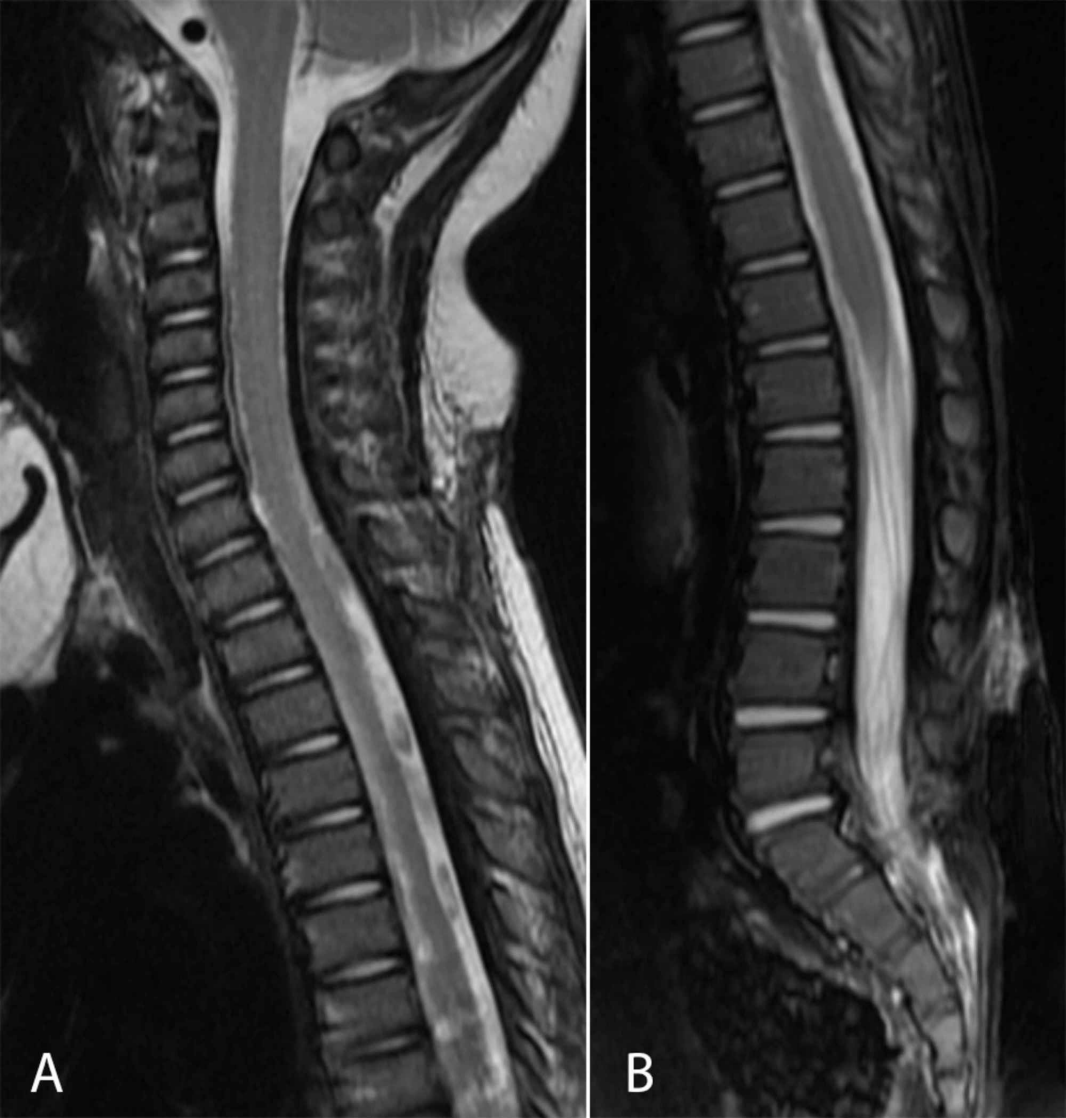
Postoperative T2 MRI of the cervicothoracic (A) and lumbosacral (B) regions with resolution of the previously noted epidural abscess
